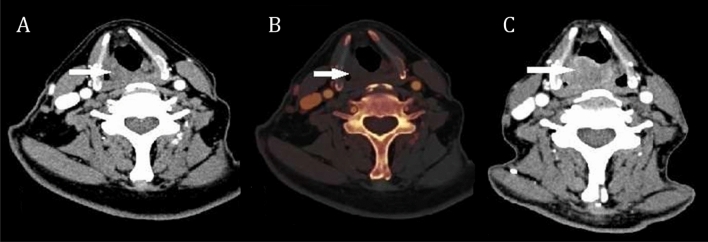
A representative patient with progressive hypopharyngeal cancer. (A) Axial CT image of arterial phase before chemotherapy, showed a tumor at the right piriform fossa but no obviouly enhancement. (B) The iodine map of arterial phase before chemotherapy, there was no obvious color in the lesion which implied a low iodine-related attenuation (IRA_mean_ 16.7HU, IRA_max_ 22.1HU). (C) Followup CT image after chemotherapy, showed that the tumor at right piriform fossa was significantly enlarged.
